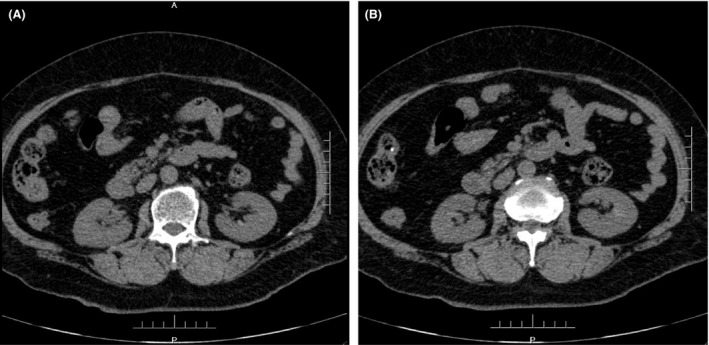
(A and B) Postoperation CT (1 month later).
